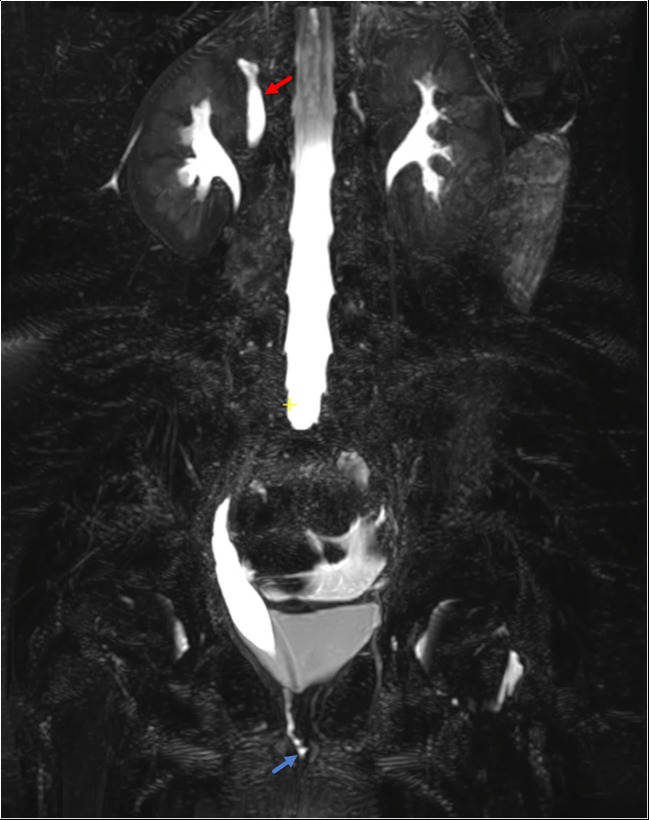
Six-year-old female with history of continuous urinary incontinence. Coronal 3D T2-weighted image demonstrates dilation of the single calyx right upper pole moiety (red arrow). The distal ureter is mildly dilated and demonstrates tapering at the level of the perineum and ultimately is seen draining at the level of the introitus (blue arrow)
